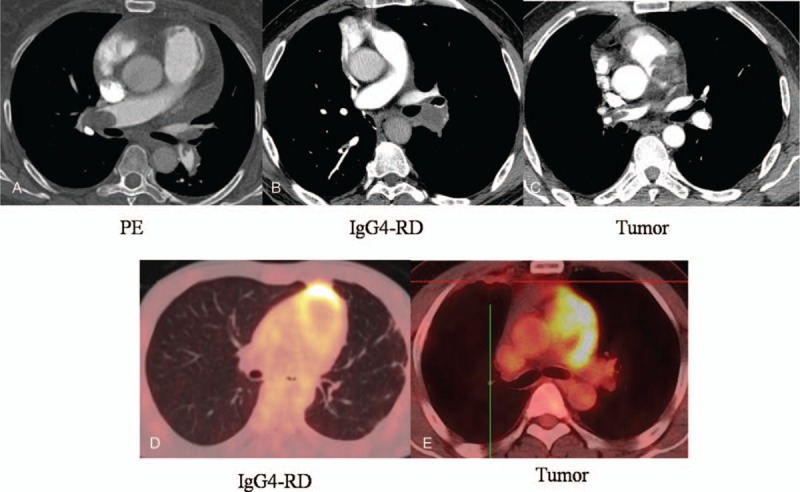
Imaging features to identify pulmonary artery IgG4-RD from PE and tumor. CTPA showed that the lesions (A and B) were not enhanced and the lesion (C) was obviously elevated. PET-CT demonstrated slight SUV intake (2.4) in the lesion (D), but obviously SUV intake (15.3) in the tumor lesion (E). CTPA = computed tomography pulmonary angiography, IgG4-RD = IgG4-related disease, PET/CT = positron emission tomography/computed tomography, SUV = standard uptake value.
